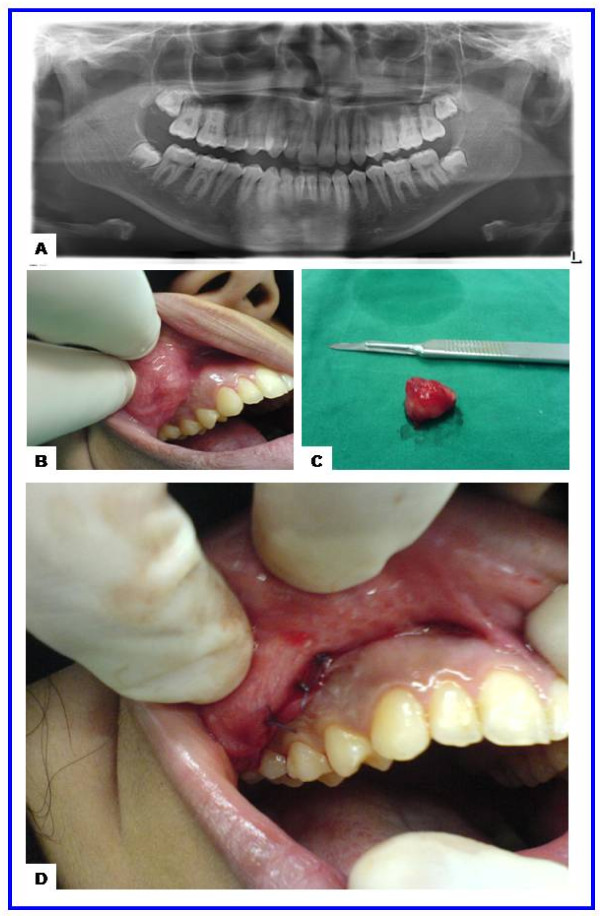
The radiological and gross features of juvenile pleomorphic adenoma of the cheek. (a) Panoramic radiograph showing unremarkable maxilla, mandible and teeth. (b) Preoperative view showing ovoid nodule in the right cheek covered by intact mucosa with congested blood vessels. (c) Gross features with a well circumscribed ovoid mass measuring 2.0 × 1.7 × 1.5 cm. (d) Postoperative view showing the site of the wound and sutures.
